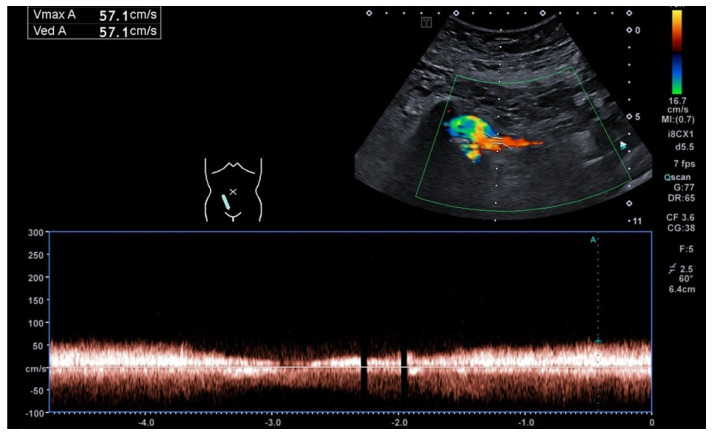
Pulse Wave Doppler (PWD) of the renal transplant vein graft shows continuous flow up to 57 cm/s.
